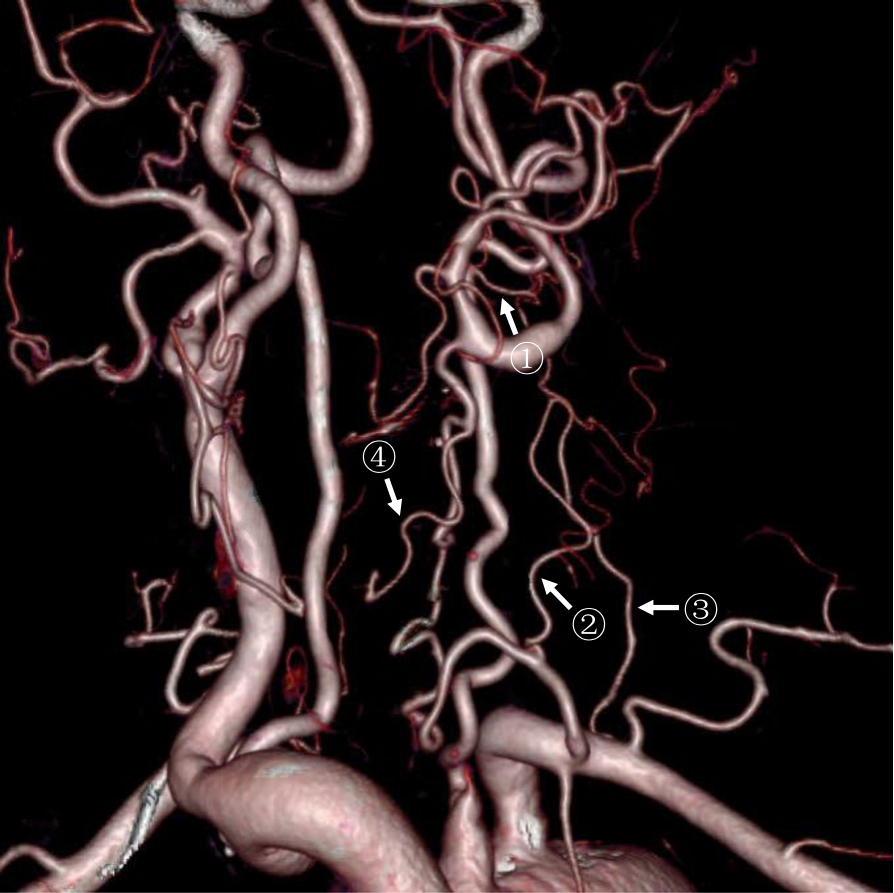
CTA discovered a fourth collateral circulation pathway that supplied ipsilateral ICA after CCAO. CTA showed coexistence of several collateral circulation pathways in one patient. Pathway 1: ipsilateral vertebral artery – occipital artery (arrow ^①^) – ECA – ICA. Pathway 2a: ipsilateral thyrocervical trunk – ascending cervical artery (arrow^②^) – occipital artery – ECA – ICA. Pathway 2b: ipsilateral costocervical trunk – deep cervical artery (arrow ^③^) – occipital artery – ECA – ICA. Pathway 4: ipsilateral thyrocervical trunk – inferior thyroid artery – superior thyroid artery (arrow ^④^) – ECA – ICA
